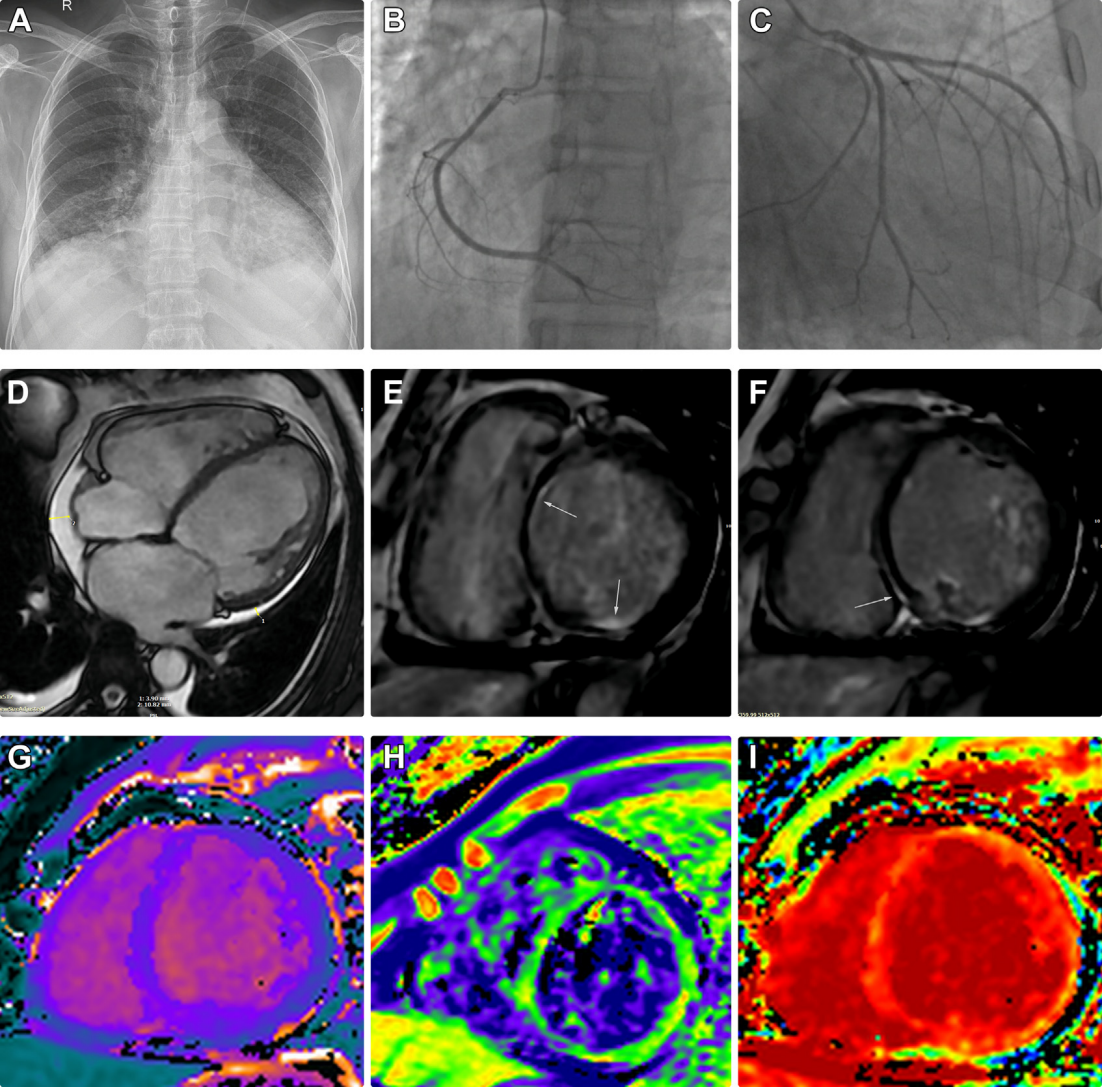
Chest X-Ray, Coronary Angiography, and Cardiac Magnetic Resonance Imaging Findings (A) Posteroanterior chest X-ray demonstrating an increased cardiothoracic ratio (>0.6). (B and C) Invasive coronary angiography revealing nonsignificant stenosis in the right and left coronary arteries. (D-I) Cardiac magnetic resonance imaging findings. (D) Four-chamber view showing dilation of all 4 cardiac chambers and a small pericardial effusion. (E and F) Late gadolinium enhancement in the subendocardial region of the interventricular septum and basal-to-mid inferior wall, with midmyocardial enhancement in the midseptal region. The total fibrosis burden was 9 g, accounting for 7% of the total left ventricular mass. (G) Increased native T1 time (1,010 ms). (H) Increased native T2 time (63 ms). (I) Increased extracellular volume fraction (45%).
